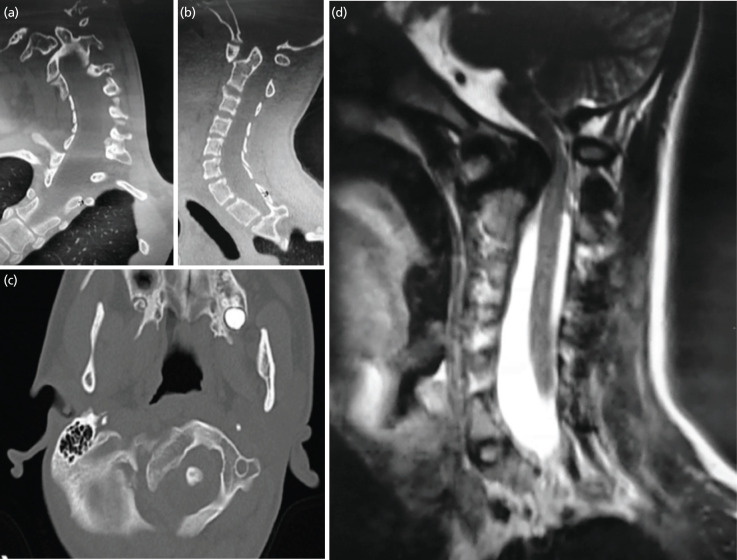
CT of the cervical spine with coronal (a), sagittal (b) and axial (c) reconstruction and MRI of the cervical spine in sagittal T2-weighted (d). CT demonstrates narrowing of the spinal canal of 50% with atlantoaxial dense interval (ADI) of 9mm, borderline basilar invagination where the tip of the odontoid process just touches the McRae line and type III atlantoaxial dislocation. MRI reveals an intramedullary high signal intensity at the region of compression indicating myelomalacia. The spinal canal is severely narrowed that the normal CSF anterior and posterior to the spinal cord is compromised.
